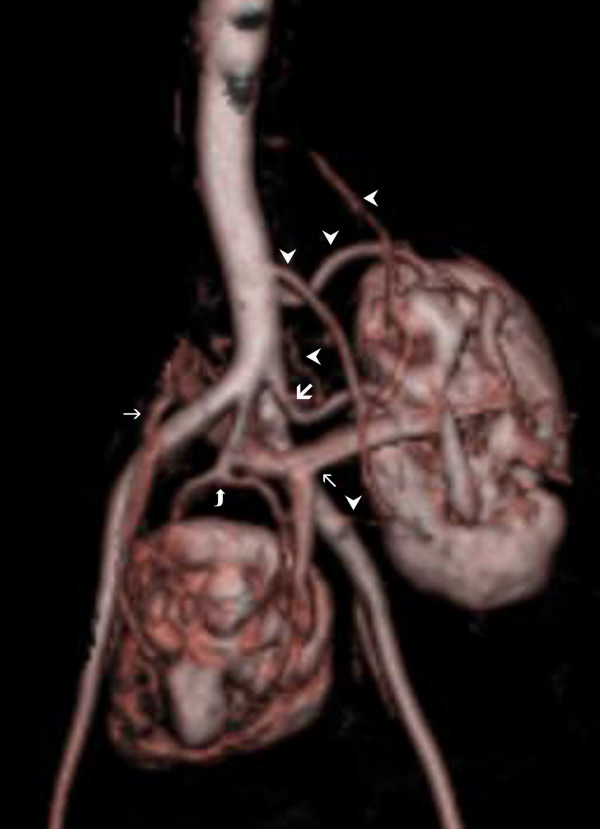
MRI contrast angiography (3D FLASH) "volume rendering" image. Accessory artery originating from iliac bifurcation of left kidney (thick arrow) and 5 polar (capsular) arteries can be seen (arrow heads). Most superior polar artery originating from distal SMA, courses through lower pole of left kidney. A renal artery branching from right common iliac artery starting point, divides into 2 branches and reaches renal pelvis (bended arrow). No polar artery is seen in this kidney. The kidney in the mid level has 2 renal veins, and the left vein is joining with the left renal vein and opening into vena cava interior (thin arrow).
